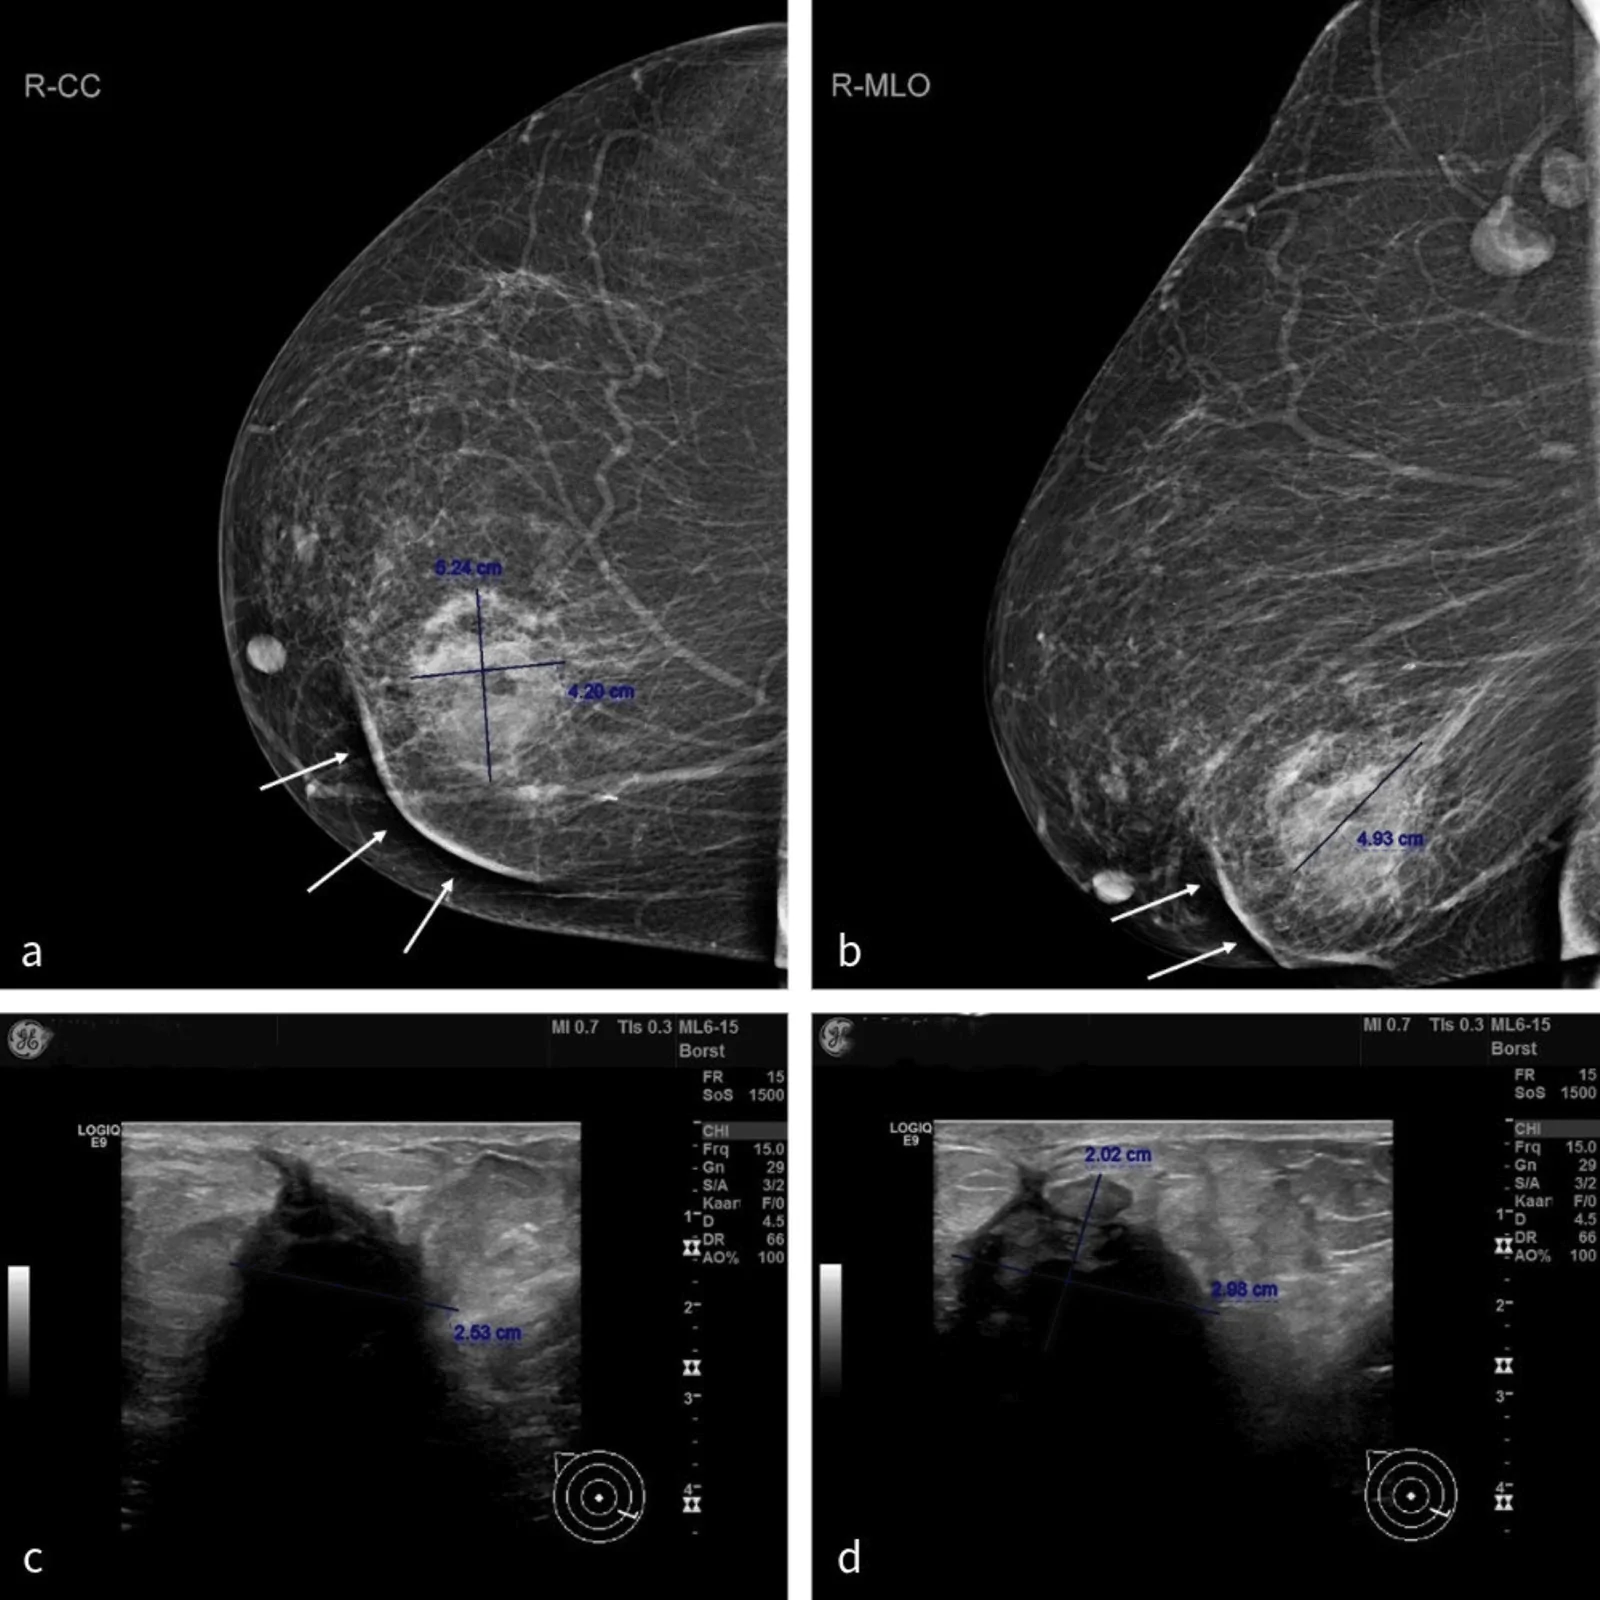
Mammography performed two months later showed an unchanged ill-defined mass measuring 6.2 × 4.2 × 4.9 cm in the inferomedial right breast (measurements, A–B) with persistent skin retraction (arrows, A–B). Ultrasound of the right breast revealed an ill-defined heterogeneous mass with posterior acoustic shadowing (C–D) (BI-RADS IV). BI-RADS: Breast Imaging Reporting and Data System
